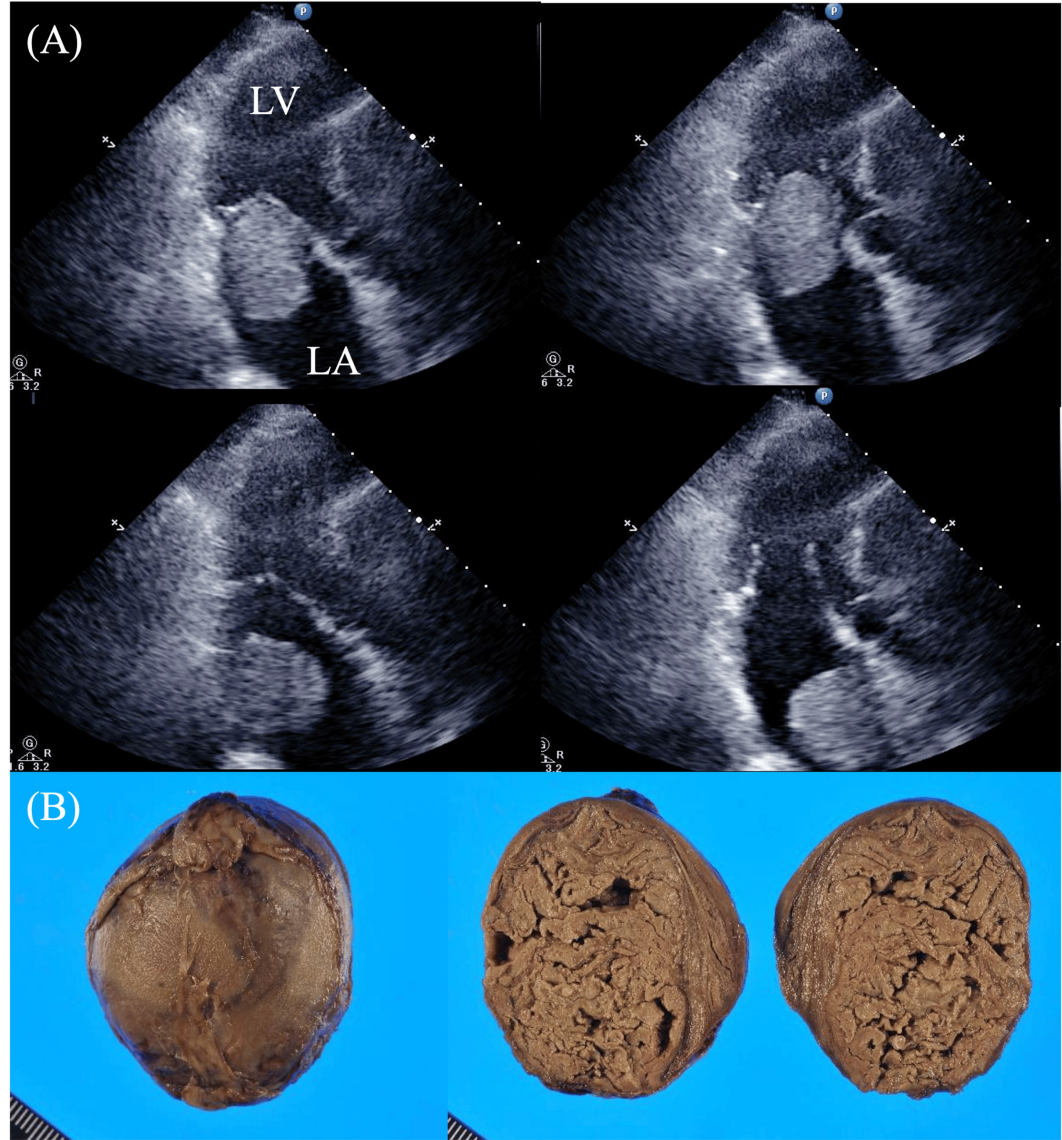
(A) UCG on admission revealed a spherical, freely mobile mass within the LA. (B) Autopsy identified a smooth-surfaced ball thrombus in the LA, about 40 mm in diameter (Case 3). UCG: ultrasound cardiography, LA: left atrium, LV: left ventricle.
